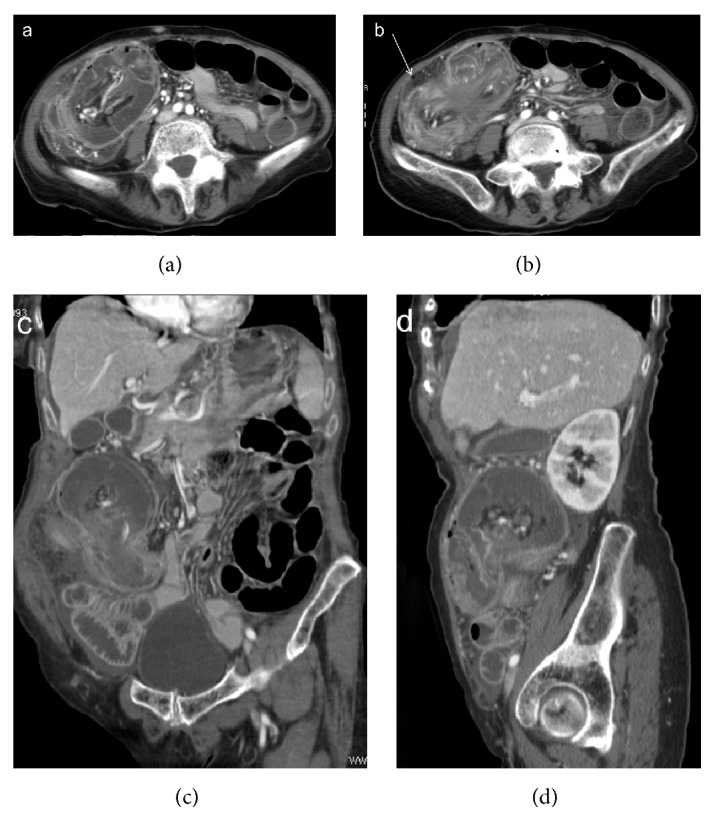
Enterocolic (ileocaecocolonic) intussusception due to a caecal carcinoma. CT images on axial scans (a, b) and oblique reformatting (c, d) show lymph nodes and vascular engorgement in the intussuscepted mesentery and fluid distention of the intussuscipiens. Extraparietal air indicates local perforation (arrow).
